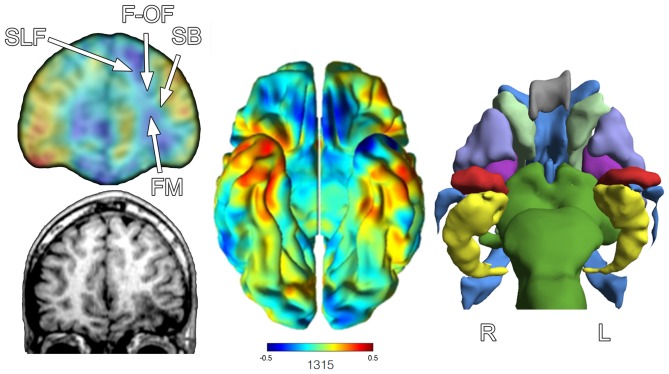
The image on the left shows at a coronal slice level the Jacobian warping to fit the TBI patient brain to the normative control template. An actual coronal T1-weighted slice at an approximate level from the anatomical MRI is presented in the lower left. The arrow points to the region of encephalomalacia. The middle image is the full Jacobian analysis depicting the ventral surface of the brain where the degree of “blueness” demonstrates where volume loss has occurred, where oppositely warm colors reflect increase. The image to the right is from FreeSurfer output to generate a 3-D image of various ROIs: red = amygdala, yellow = hippocampus, purple = putamen, gray = corpus callosum, light green = caudate, blue = ventricle, violet = globus pallidus, darker green = brainstem. Note that the left hippocampus, amygdala and putamen are smaller in appearance, empirically confirmed based on FreeSurfer volumetric findings in reference to an age- and demographically-matched control sample. R, right, L, left. White arrows in the upper left image depict general location of four major fasciculi that interface with the frontal lobe. SLF, Superior Longitudinal Fasciculus; F-OF, Fronto-Occipital Fasciculus; SB, Striatal Bundle; FM, Forceps Minor.
